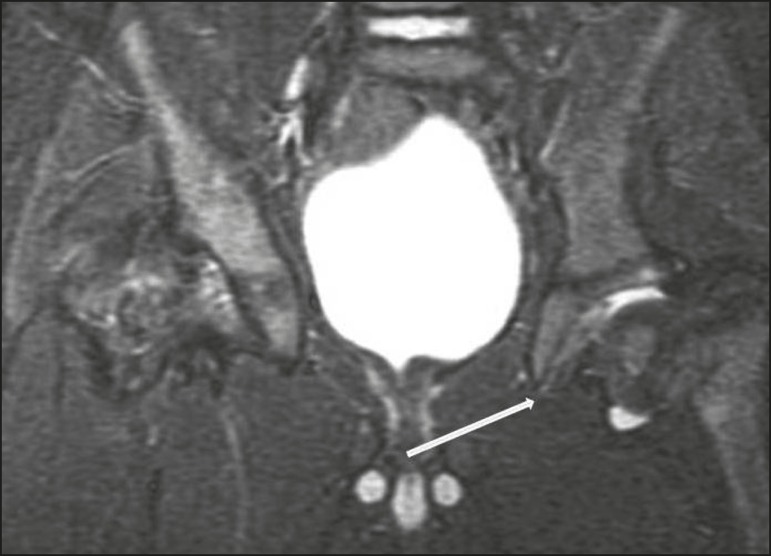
MRI of the hip. STIR coronal sequence showing signs of pronounced epiphysiolysis. Note the moderate joint effusion.
